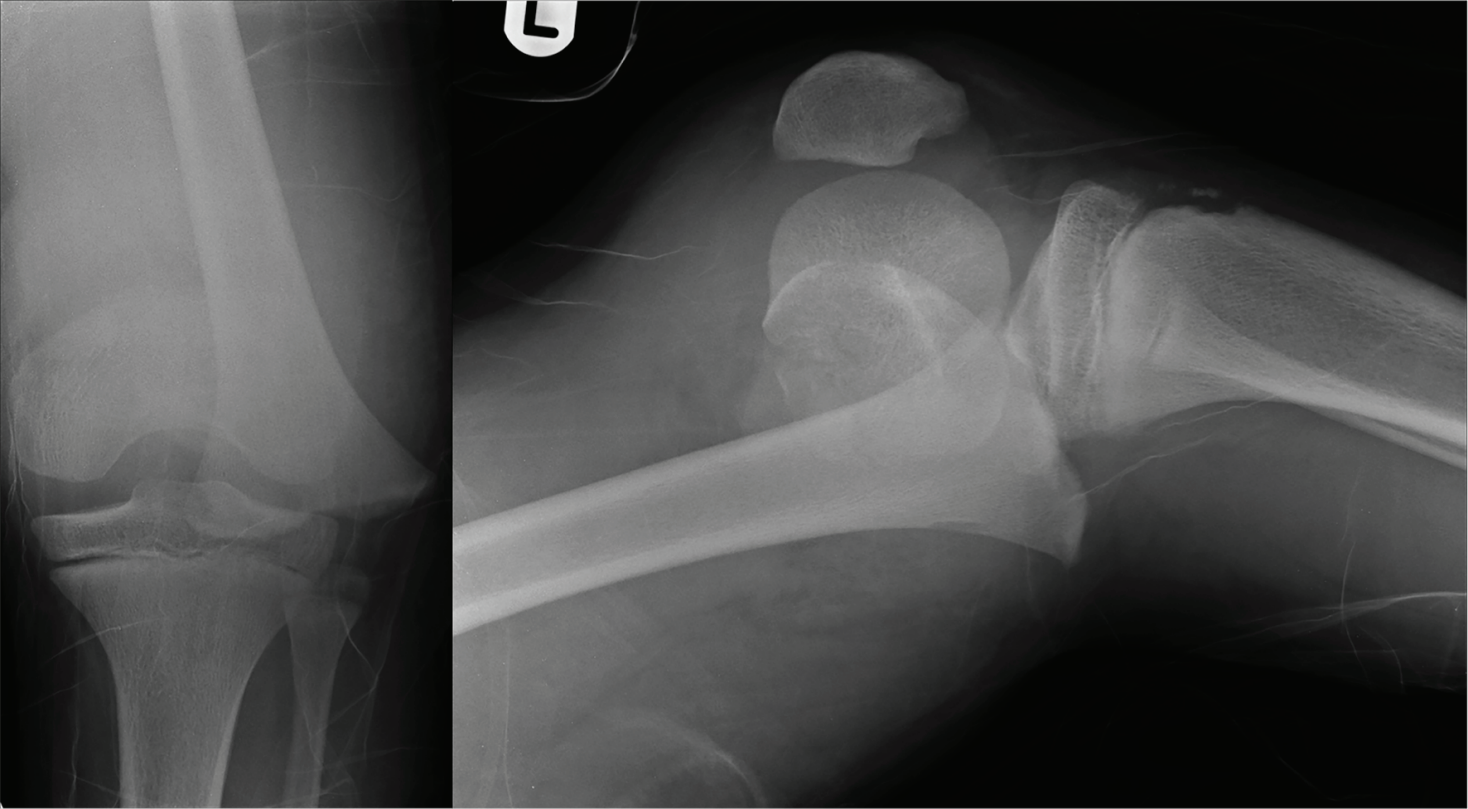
Plain antero-posterior and lateral radiographs demonstrating a fracture through the distal femoral physis with complete anterior displacement
